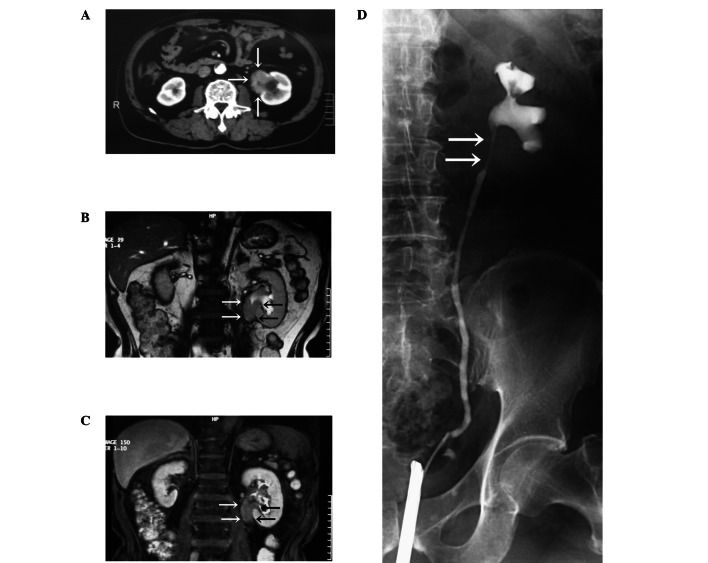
Imaging studies. (A) Abdominal computed tomography (CT) revealing a left hydronephrosis and diffuse pelvic wall thickening (arrows) that are enhanced slightly by contrast media. (B) T2-weighted magnetic resonance imaging (T2WI-MRI) demonstrating a mass-like lesion (arrows) surrounding the renal pelvis and upper ureter, whose signal intensity is slightly lower than that of the renal parenchyma. (C) Gadolinium-enhanced MRI reveals a poorly enhanced pyeloureteral junction with a thickened wall (arrows). (D) Retrograde pyelograpy shows a >3 cm narrowing of the upper ureter (arrows), and the surface of this area appears to be smooth.
